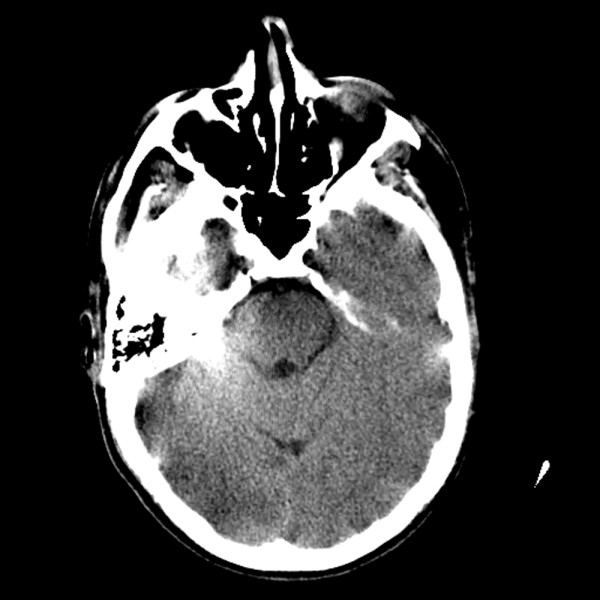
A head CT without contrast demonstrating subtle, ill-defined regions of low attenuation in the bilateral temporo-occipital lobes.
